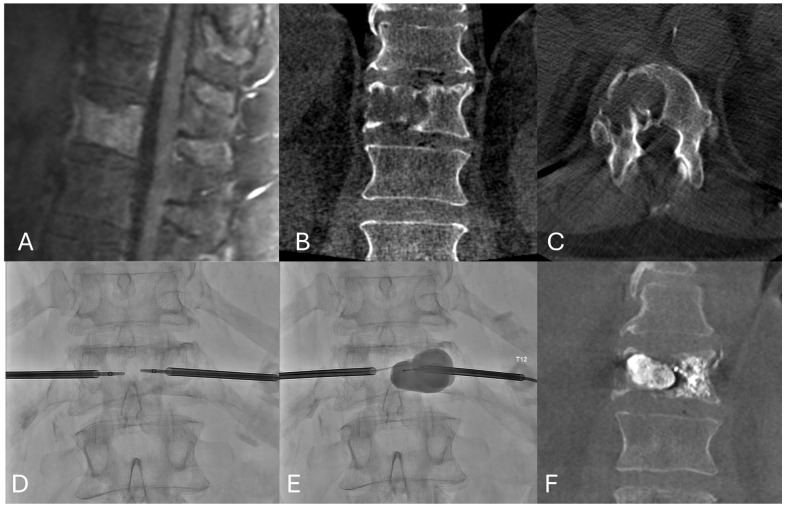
Palliative pain relief and structural support. A 72-year-old male with 3-year history of metastatic renal cell cancer presented with new onset of lower thoracic back pain. Radiation was performed, but due to the large lytic defect and ongoing pain limiting his activities of daily living, thermal ablation and kyphoplasty were performed. (A) Sagittal Post Gadolinium T1 weighted image demonstrating a pathologic fracture without epidural disease or retropulsion. (B,C) Coronal and Axial CT demonstrate more than 50% of the T12 vertebral body replaced by lytic metastasis. (D) AP fluoroscopic image demonstrating radiofrequency ablation using 20 mm bilateral Osteocool probes. (E) A 20 mm curved kyphoplasty balloon and 10 mm straight kyphoplasty balloon fill the lytic defect and are kept inflated during the left-sided PMMA cement filling to prevent extravasation, and finally deflated, and the lytic cavity is filled. (F) Coronal CT demonstrating results of post-RF ablation kyphoplasty.
